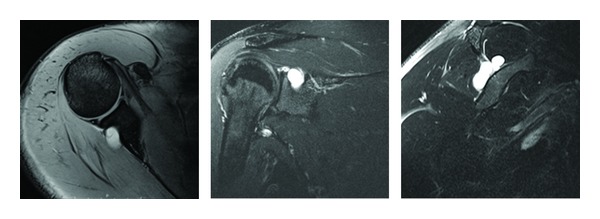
Axial, coronal, and sagittal MRI images of a patient with multiple paralabral cysts in the suprascapular notch and spinoglenoid notch.
